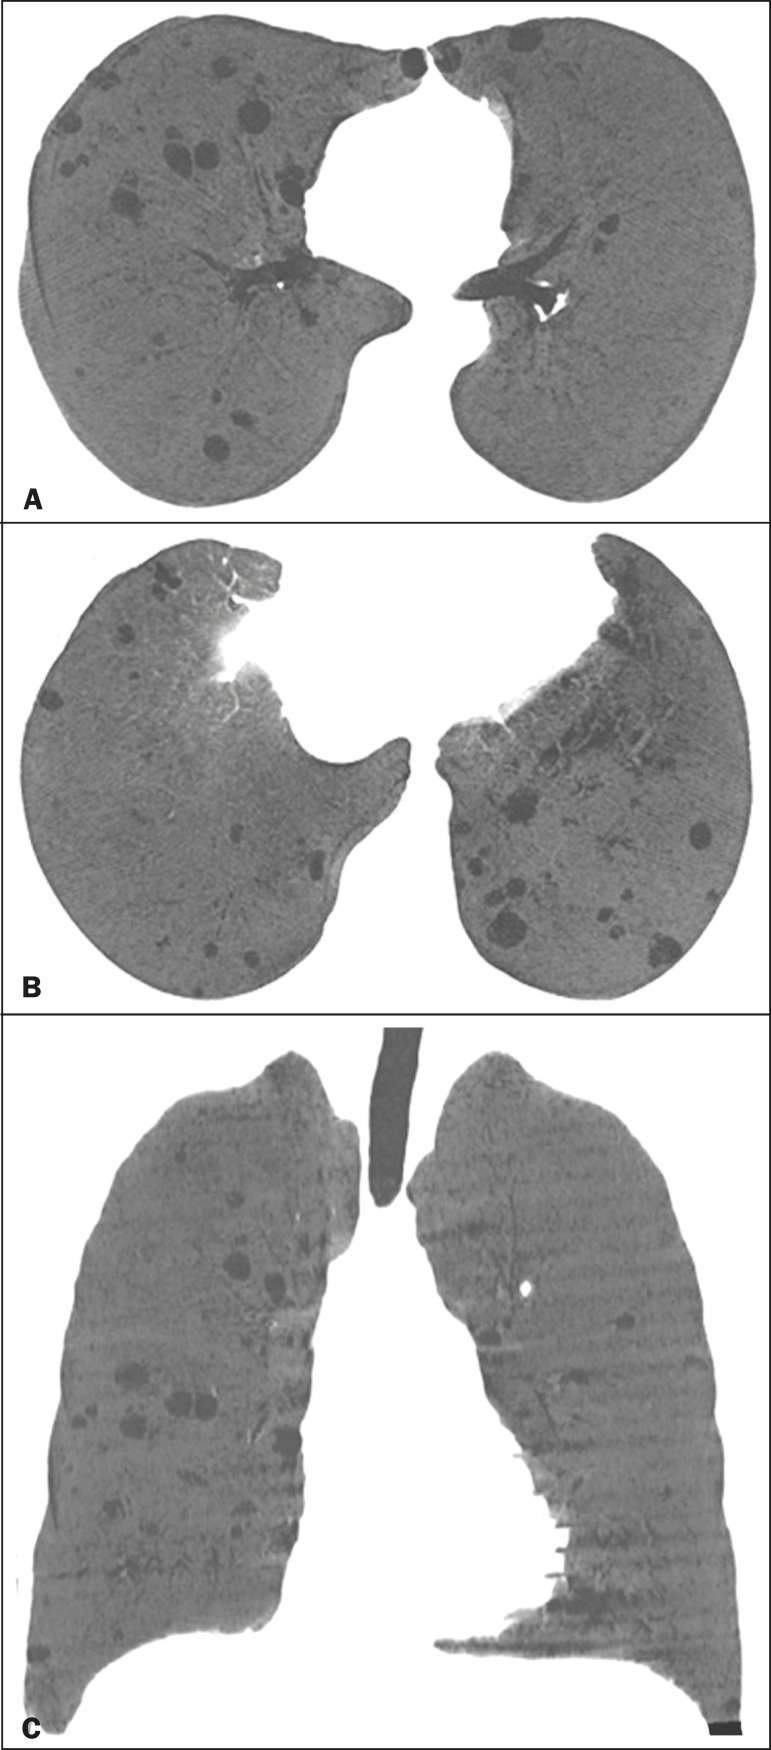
A 64-year-old woman with LIP related to Sjögren syndrome. Chest CT scans obtained with minimal-intensity projection with axial (A,B) and coronal (C) reconstructions show multiple bilateral pulmonary cysts with a random distribution.
